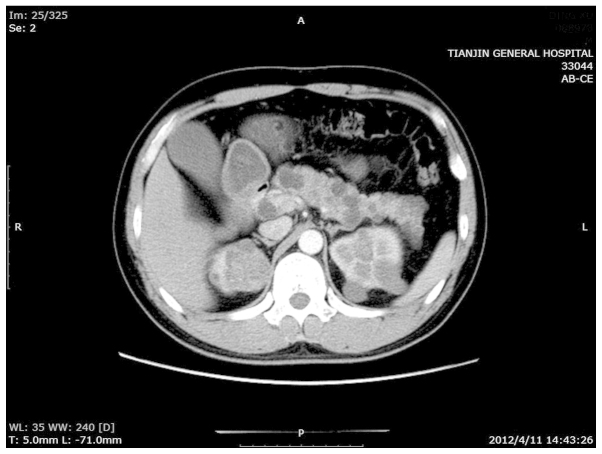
Enhanced computed tomography scan showing the lesion in the pancreas and kidneys.
